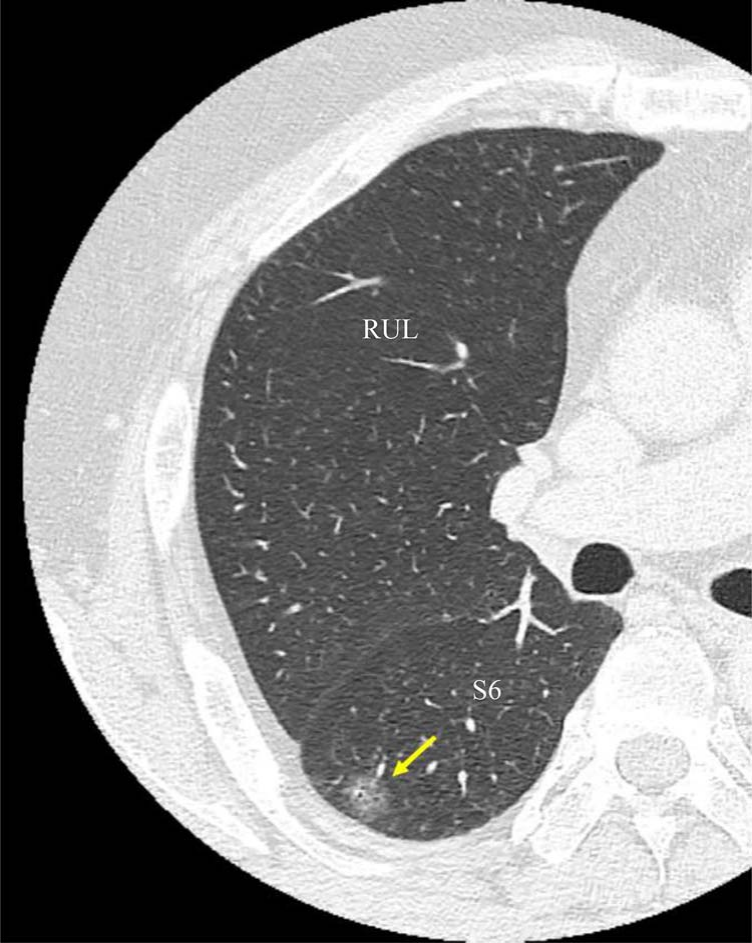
An image from CT scan taken in the axial plane. The tumor is located in the S6 of the right lung (arrow). RUL, right upper lobe; S6, superior segment.
